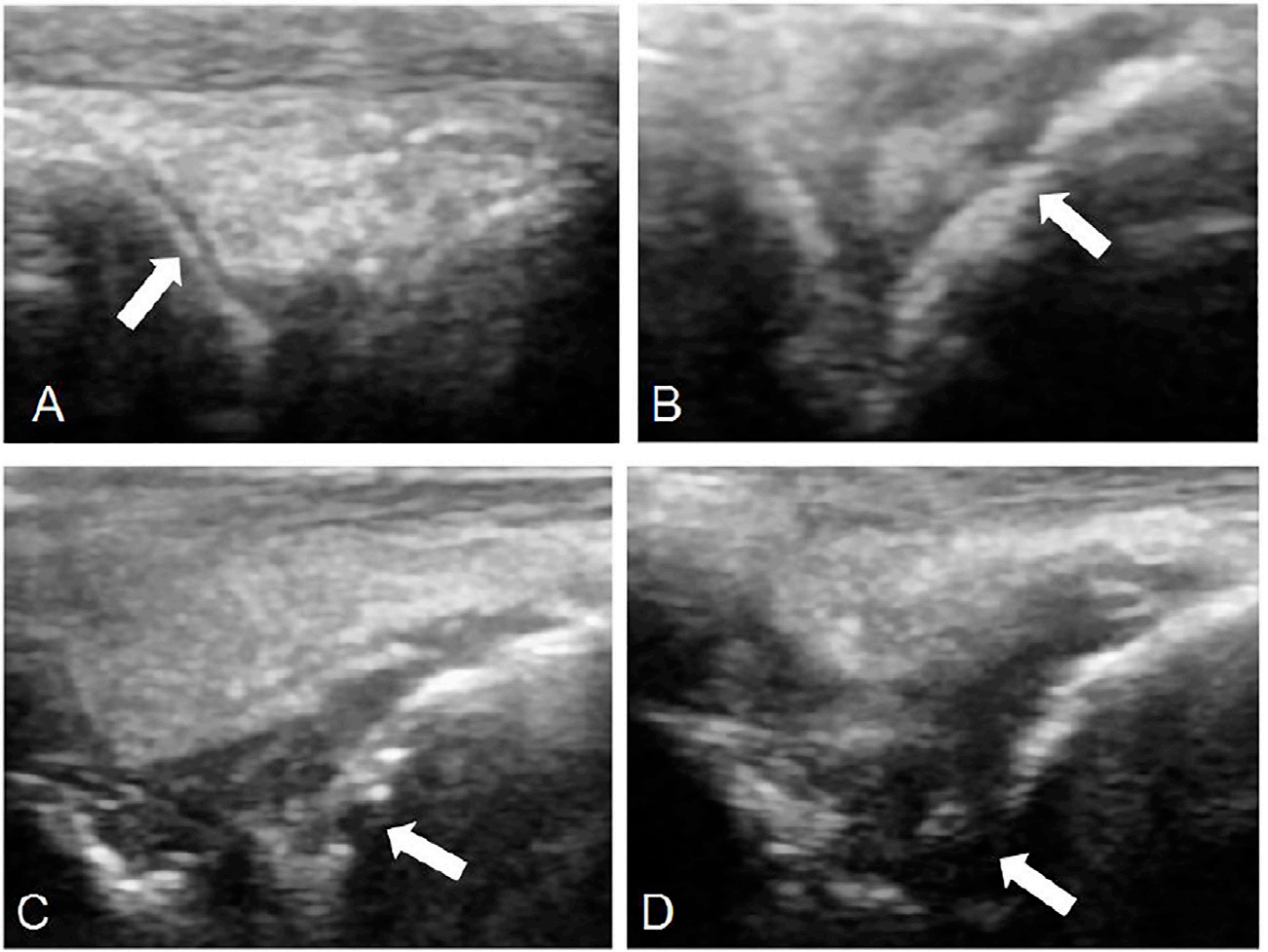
Observation of bone destruction by ultrasound. 0: No bone damage on the surface of bone (TP-SLN group, (A)). One point: The surface of bone is not smooth, but there is no bone destruction (TP group, (B)). Two points: Slight bone defect on bone surface (DMSO group, (C)). Three points: extensive bone destruction on bone surface (control group, (D)).
